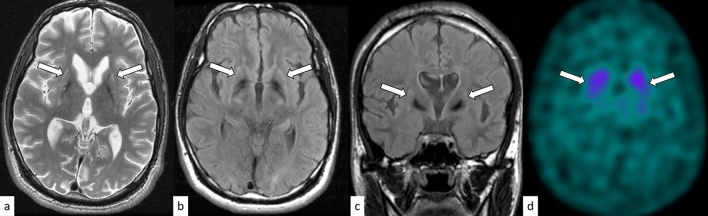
A 24-year-old man with Huntington disease. T2-weighted axial image a, FLAIR axial image b, and FLAIR coronal image c show hyperintensity and atrophy of the bilateral caudate nuclei and putamima, and atrophy of the globi pallidi (arrows). Decreased DAT uptake with a specific binding ratio of 2.12 (right striatum) and 2.09 (left striatum) is observed on DaTscan (d)
